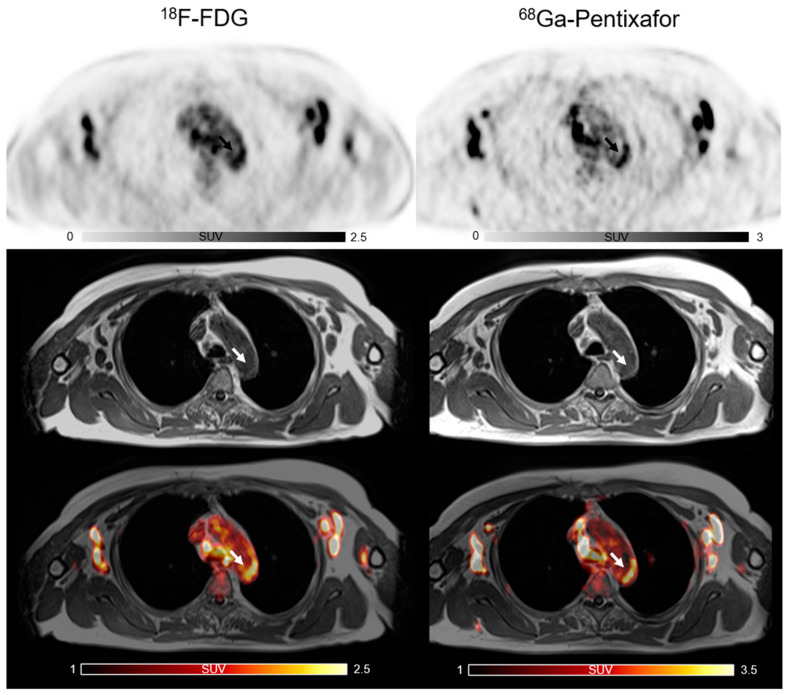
The co-localized focal vascular uptake of both [^18^F]FDG and [^68^Ga]PentixaFor (white arrow) in active atherosclerotic lesions.
